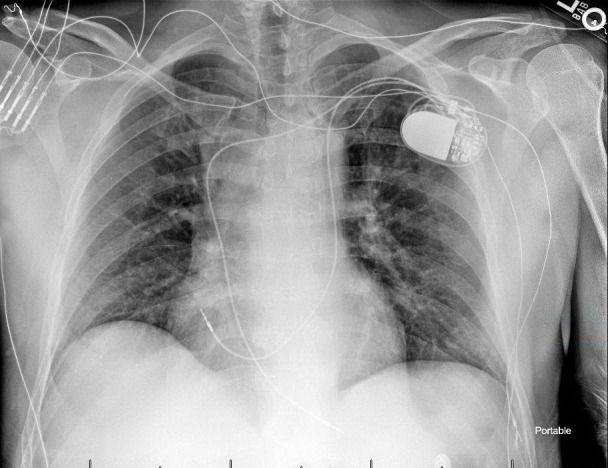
Post-implantation roentgenogram Post-procedure roentgenogram demonstrating final lead position with the right ventricular lead traversing the bridging innominate vein and the right atrial lead placed via the persistent left superior vena cava and enlarged coronary sinus.
